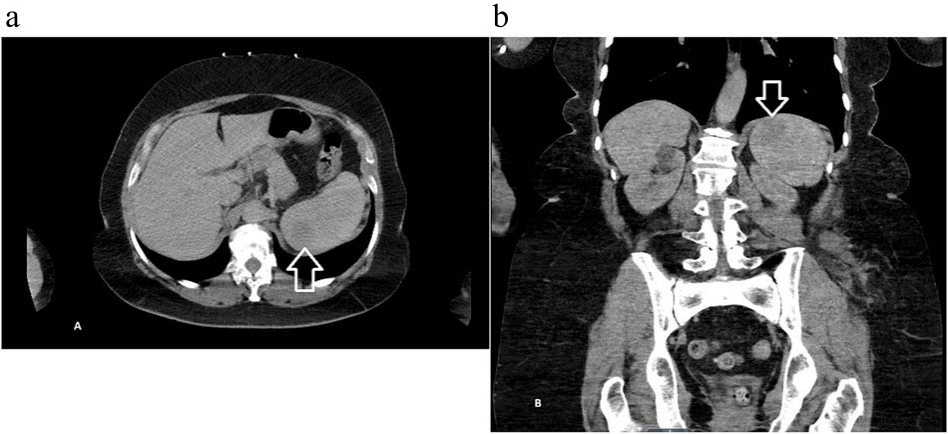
Computed tomography of the abdomen and pelvis with contrast showing an indeterminate round 3.5 cm hypo-attenuating lesion in the spleen: (a) axial and (b) coronal views with arrow marking the mass.
